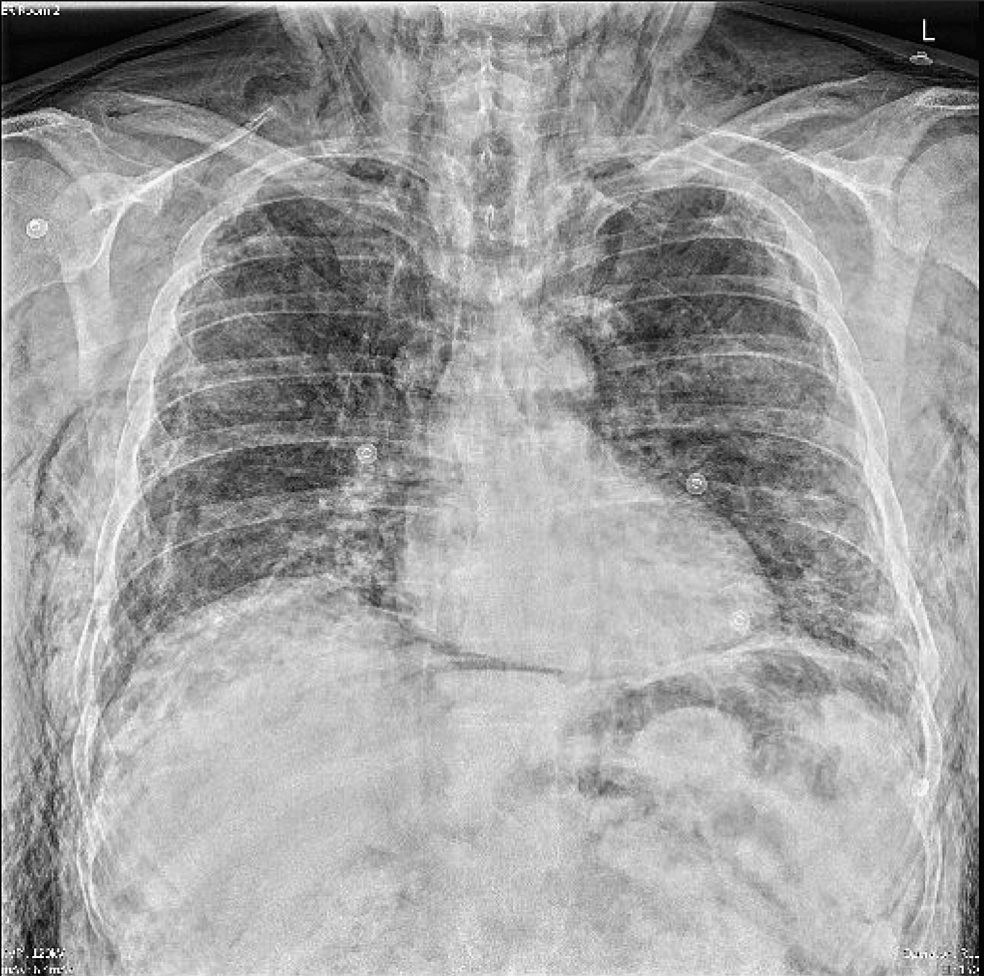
Chest X-ray demonstrating diffuse subcutaneous emphysema and pneumoperitoneum.
